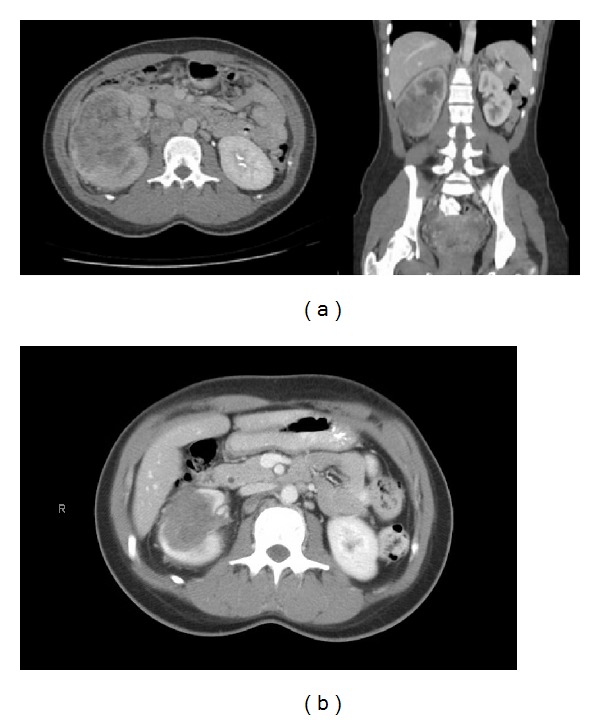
(a) CT scan of abdomen showing necrotic right-sided tumor replacing the kidney. (b) CT scan after chemotherapy.
